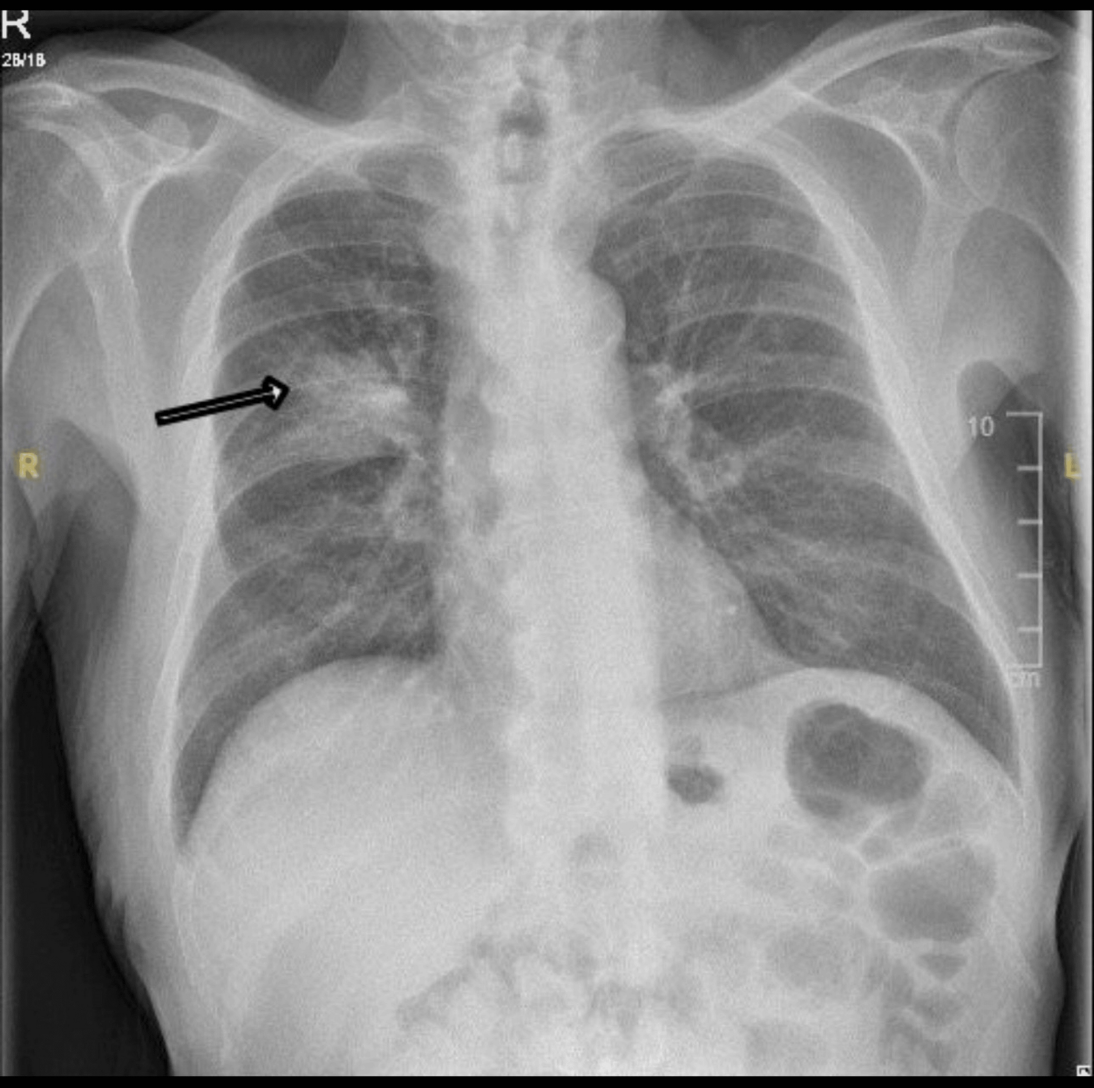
Posteroanterior chest radiography The image shows a right perihilar mass (arrow)
